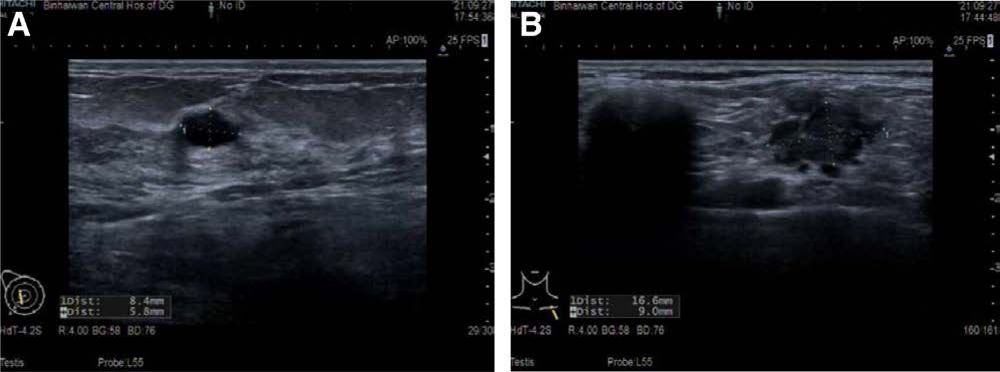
Color ultrasound of (A) a hypoechoic area in the right breast, about 8 mm × 6 mm in size, considering BI-RADS Class 4a; (B) enlarged lymph nodes in the left Cervical Region VII, approximately 17 mm × 9 mm in size.
